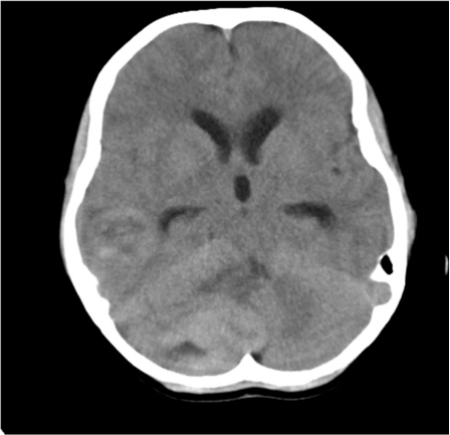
CT image of the 5-year-old boy. Two intracranial tumors were observed, a right temporal tumor and a right cerebellar tumor. The tumors had a round shape and were of high density
